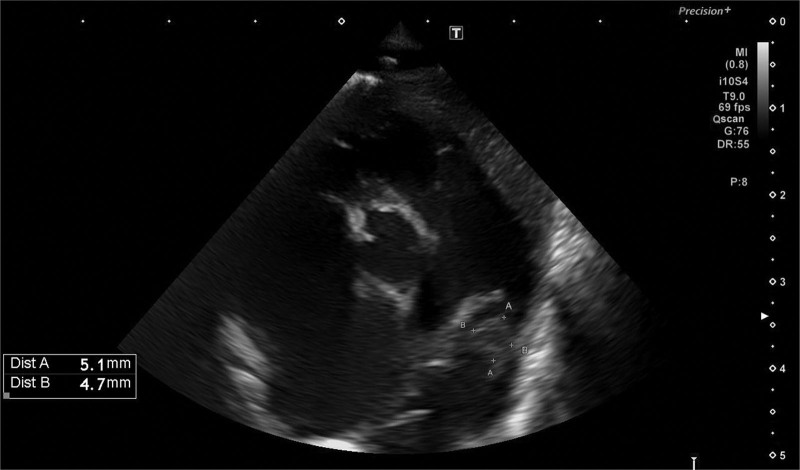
Echocardiogram (ductal view) showing the vegetation inside the ductus arteriosus.
